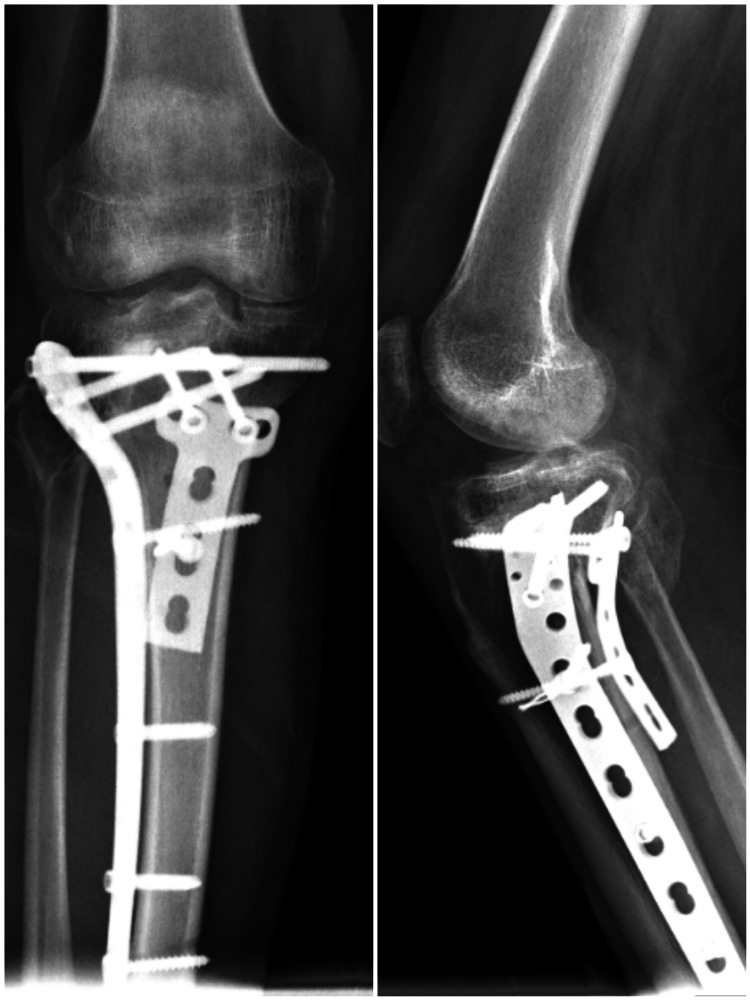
Anteroposterior and lateral radiographs of a patient who was treated using the dual locked plate due to Schatzker type VI bicondylar tibial plateau fracture
